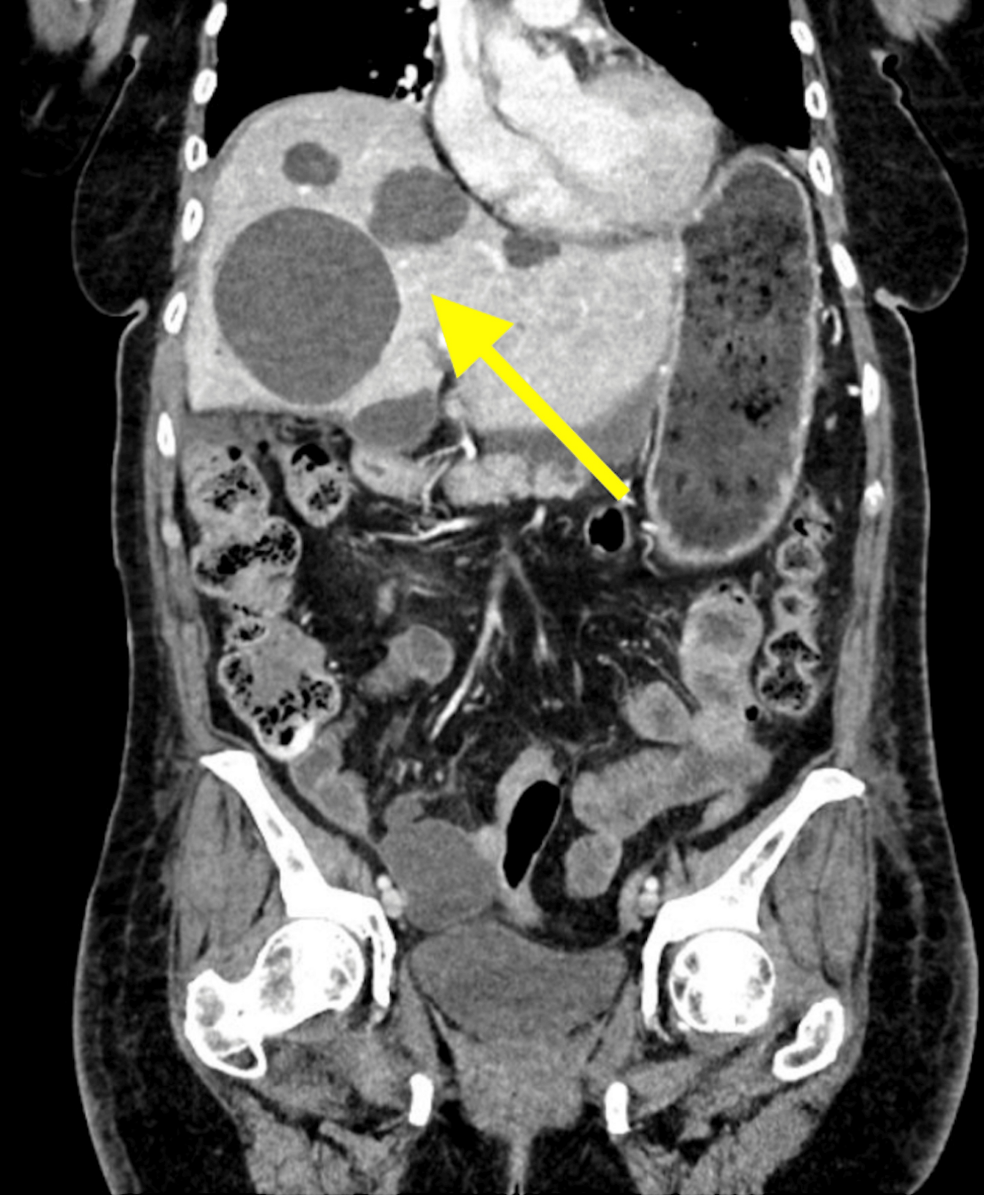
Multiple hepatic cysts indicated by a yellow arrow on CT scan CT: computed tomography
